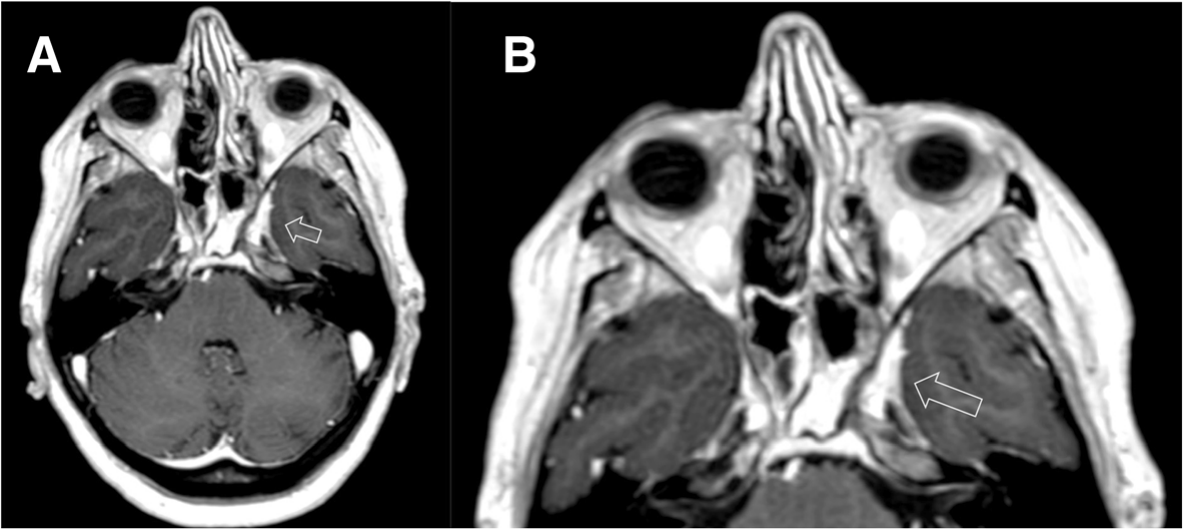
Tolosa-Hunt syndrome. MRI T1-weighted post-gadolinium axial image (a, and enlargement in b) shows enhancing inflammatory infiltration in the left orbital apex extending into the cavernous sinus (empty arrows)
